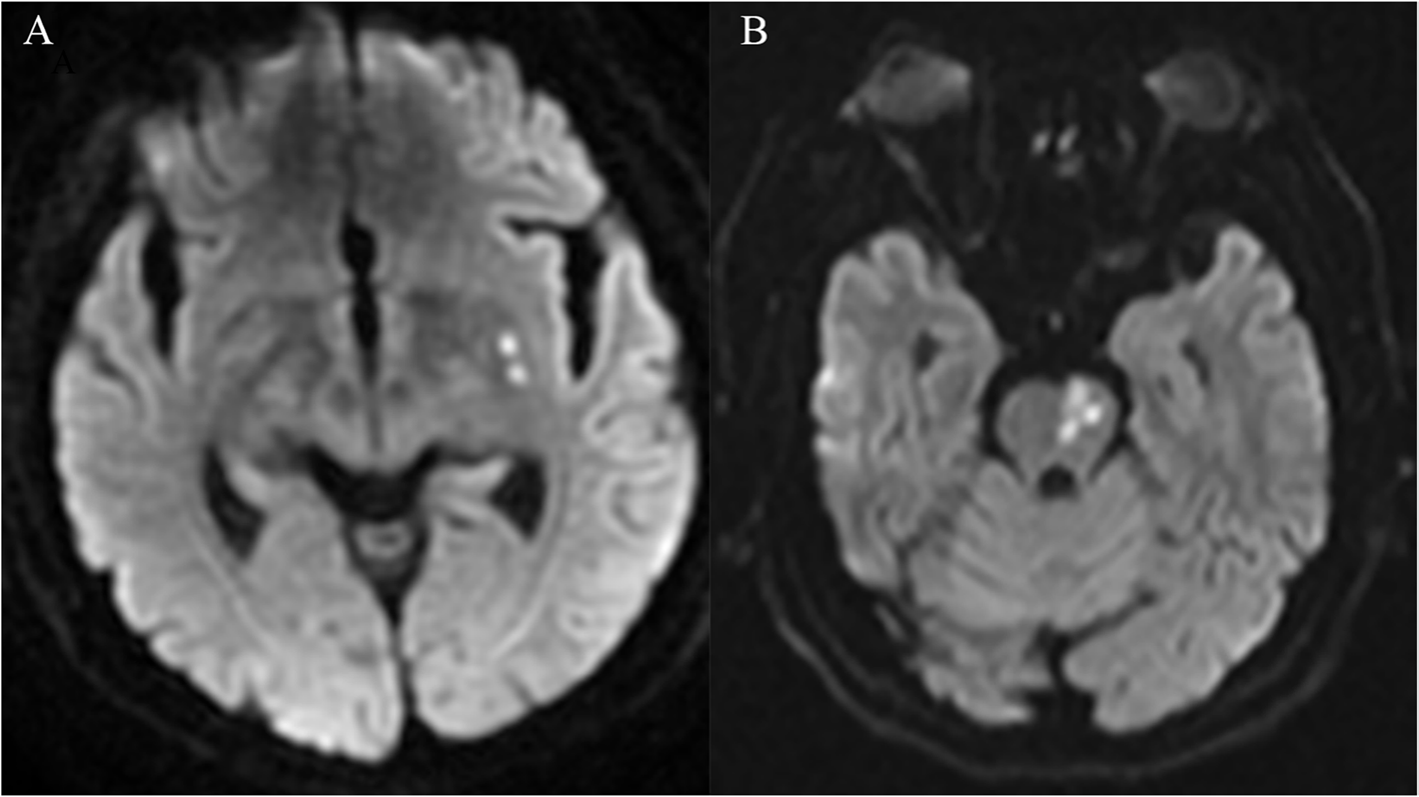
(A) Example of the “island sign”pattern on DWI in the lenticulostriate arteries (LSA) territory. (B) Example of the “island sign” pattern on DWI in the paramedian pontine arteries (PPA) territory
